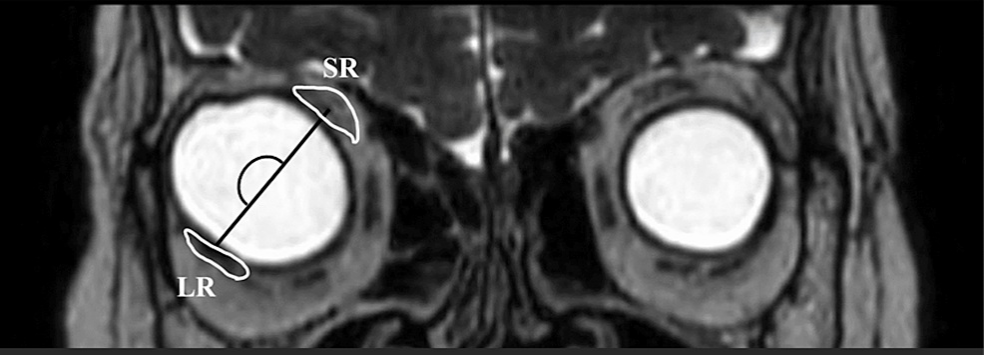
MRI image before the surgery An MRI showed that the posterior eyeball of her right eye had dislocated out of the supratemporal muscle cone. The dislocation angle between the superior rectus (SR) and lateral rectus (LR) was 181° in the right eye.
